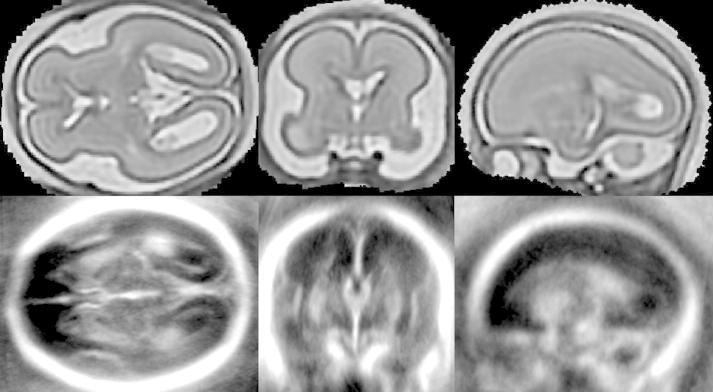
Axial, coronal and sagittal view of brain MRI of a fetus with GA 23 weeks (first row) and the average of the 27 fetal brain 3D US with GA 18–22 weeks, aligned with the MRI.
